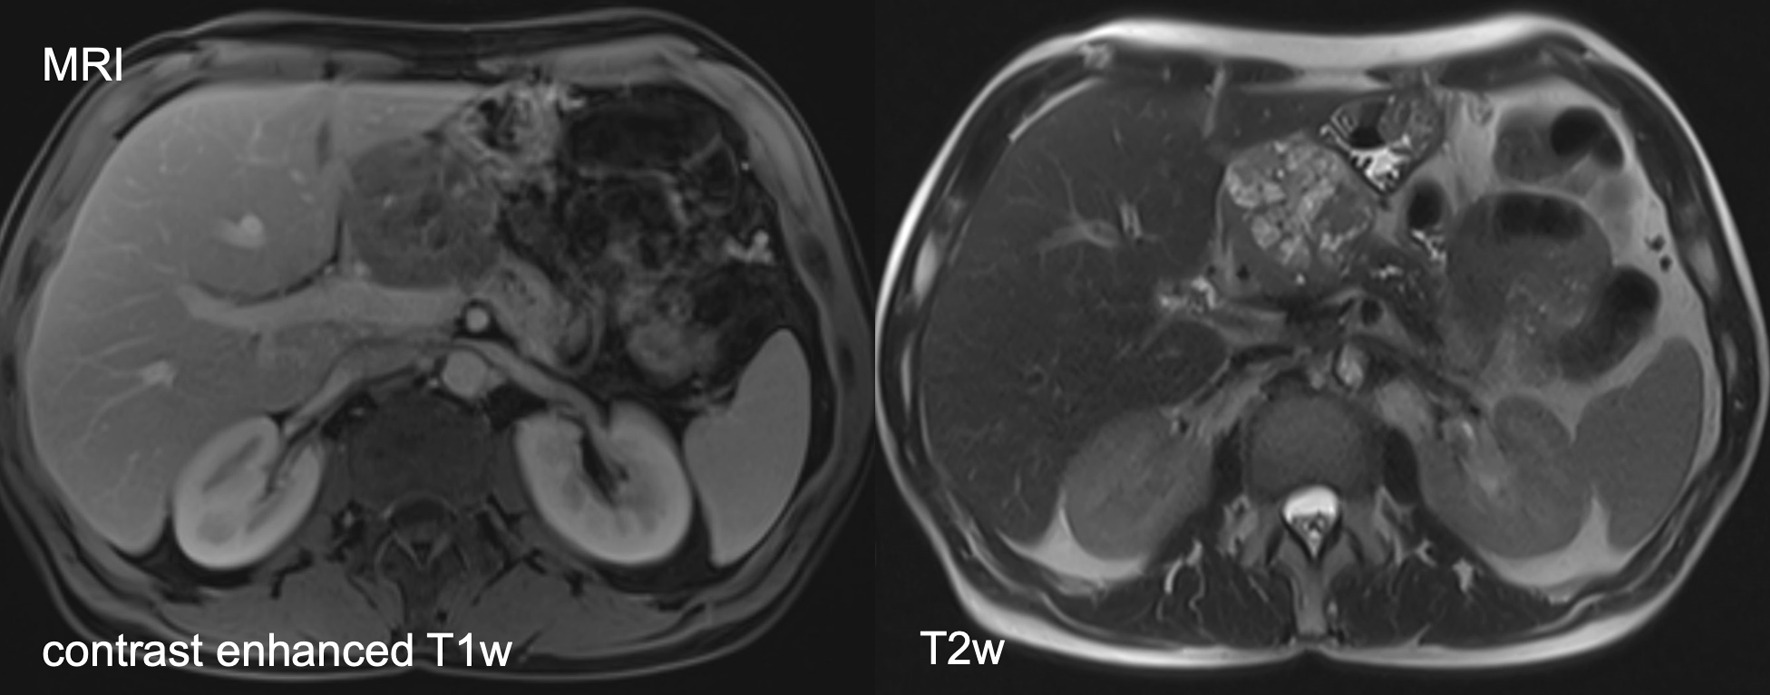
MRI revealing a 5 × 6 cm inhomogeneous polycystic mass with suspected solid components in the pancreatic head with a partly compression of the duodenum
